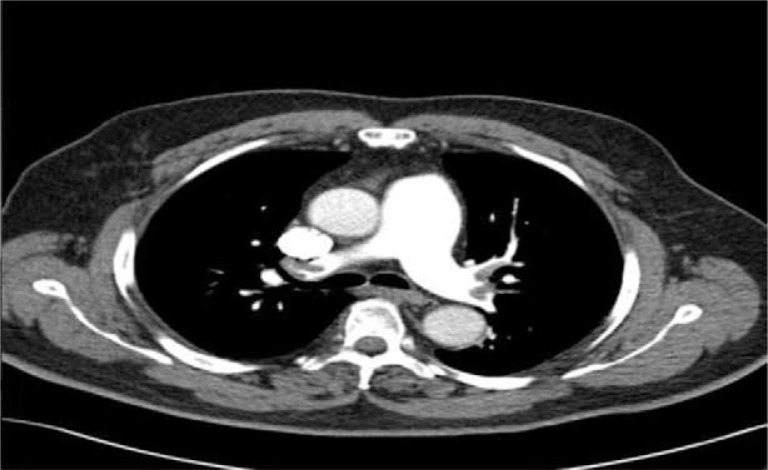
Spiral CT-scan of chest demonstrating large clot burden in the pulmonary arteries bilaterally.
